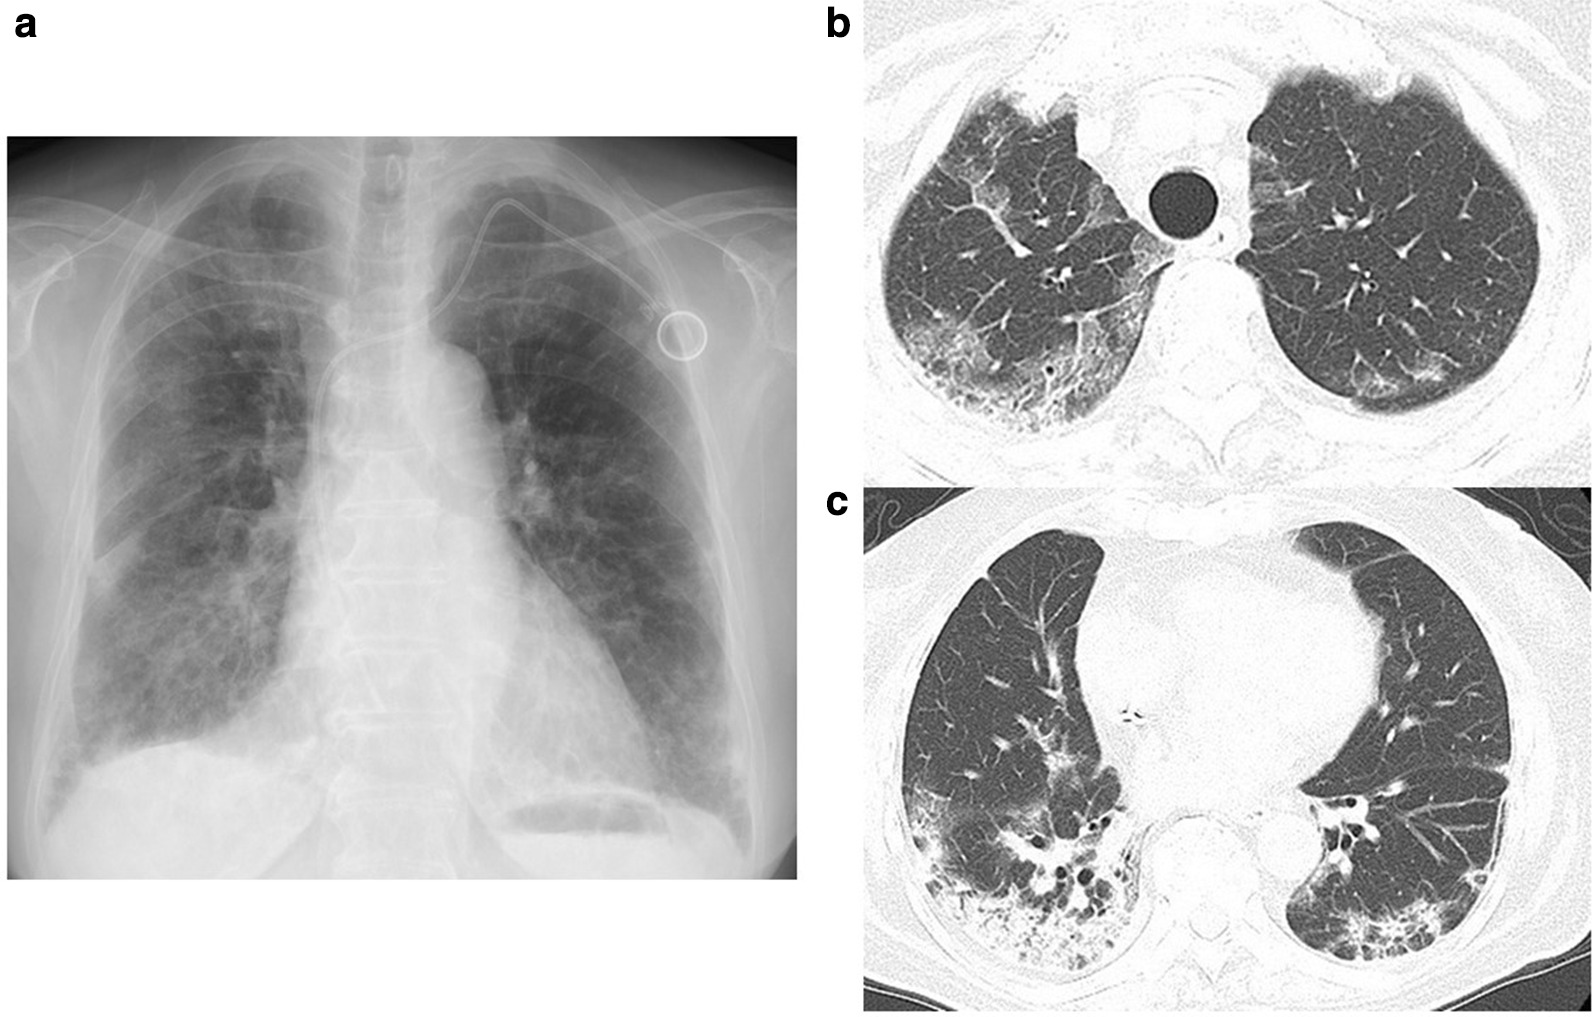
Chest x-ray and thoracic computed tomography (CT) before corticosteroid treatment. Chest x-ray (a) and thoracic CT scans (b, c) show worsening consolidation
